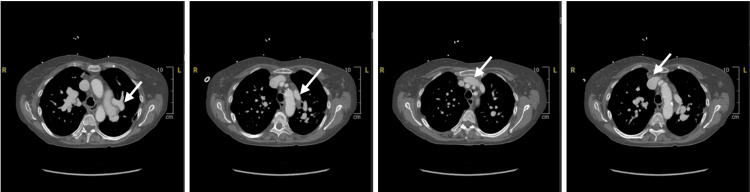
Cardiac CT of left superior pulmonary vein Cardiac CT demonstrating left superior pulmonary venous drainage into the left brachiocephalic and ultimately into the SVC (left to right). CT, computed tomography; SVC, superior vena cava.
